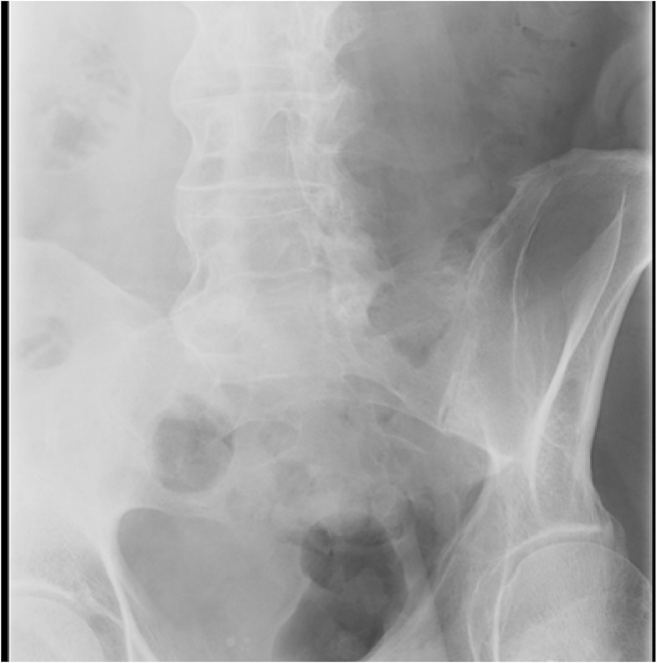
X-rays of sacroiliac joints and lumbar spine. Show partial fusion of the bilateral sacroiliac joints, lumbar spine syndesmophytes, and fusion of the posterior spinous processes known as `bamboo spine.
